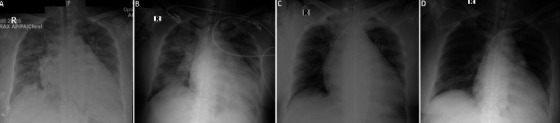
A) chest radiographs on arrival; B) intubated; C) 6 hours after intubation; D) intensive care unit day 4
